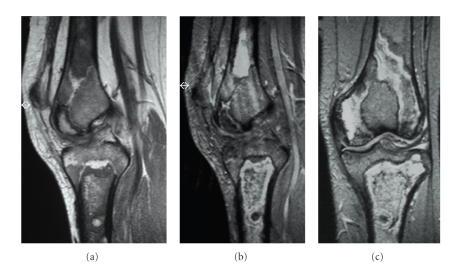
(a), (b) and (c) T1W sagittal, T2W sagittal, and coronal MRI knee in a patient with sickle cell anaemia showing, low signal change on T1W images with corresponding areas of solid and serpiginous high signal change on T2W sequence consistent with medullary infarcts involving the femur and tibia.
